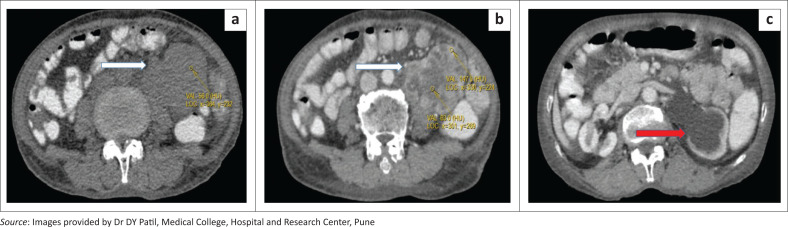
(a–c) Axial CT of abdomen showing a well defined solid retroperitoneal mass (white arrow) anterior to the left psoas and medial to the descending colon appearing isodense to muscle on pre-contrast (a), showing heterogeneous post contrast enhancement (b), causing proximal left hydronephrosis and hydroureter (red arrow) (c).
